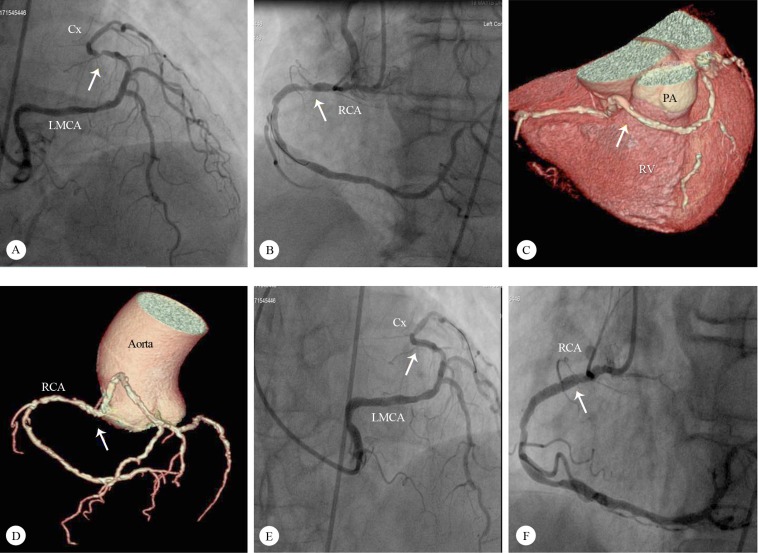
A) Left anterior oblique cranial angiographic view of the LMCA originating from the right sinus of Valsalva and a severe midportion LCx stenosis (arrow indicates the stenosis); B) Left anterior oblique angiographic view of a severe proximal RCA stenosis (arrow indicates the stenosis); C) Computed tomographic anterolateral angiographic imaging of the LMCA originating from the right sinus of Valsalva (arrow indicates the LMCA course); D) Computed tomographic anteroposterior angiographic imaging of the origin and course of the LMCA and the RCA (arrow indicates the LMCA and RCA origin); E) Left anterior oblique cranial angiographic view of the successful percutaneous intervention on the midportion of the LCx (arrow indicates the stent); F) Left anterior oblique angiographic view of the successful percutaneous intervention on the proximal RCA (arrow indicates the stent in the proximal RCA)
